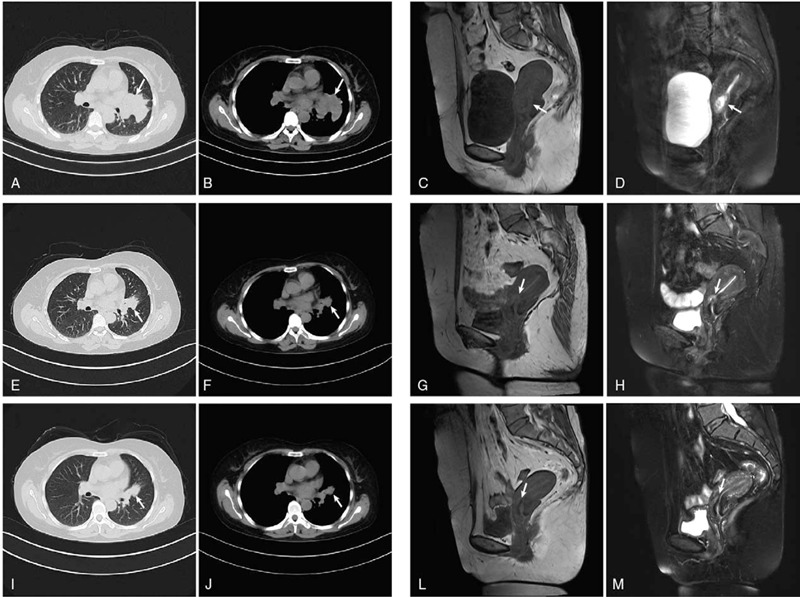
Axial CT demonstrates an irregular mass within the upper lobe of the left lung (A, B) one month (E, F) and 2 months (I, J) after treatment with osimertinib (white arrows). Sagittal MRI reveals a cervical mass lesion (C, D) one month (G, H) and 2 months (L, M) after treatment with osimertinib (white arrows).
